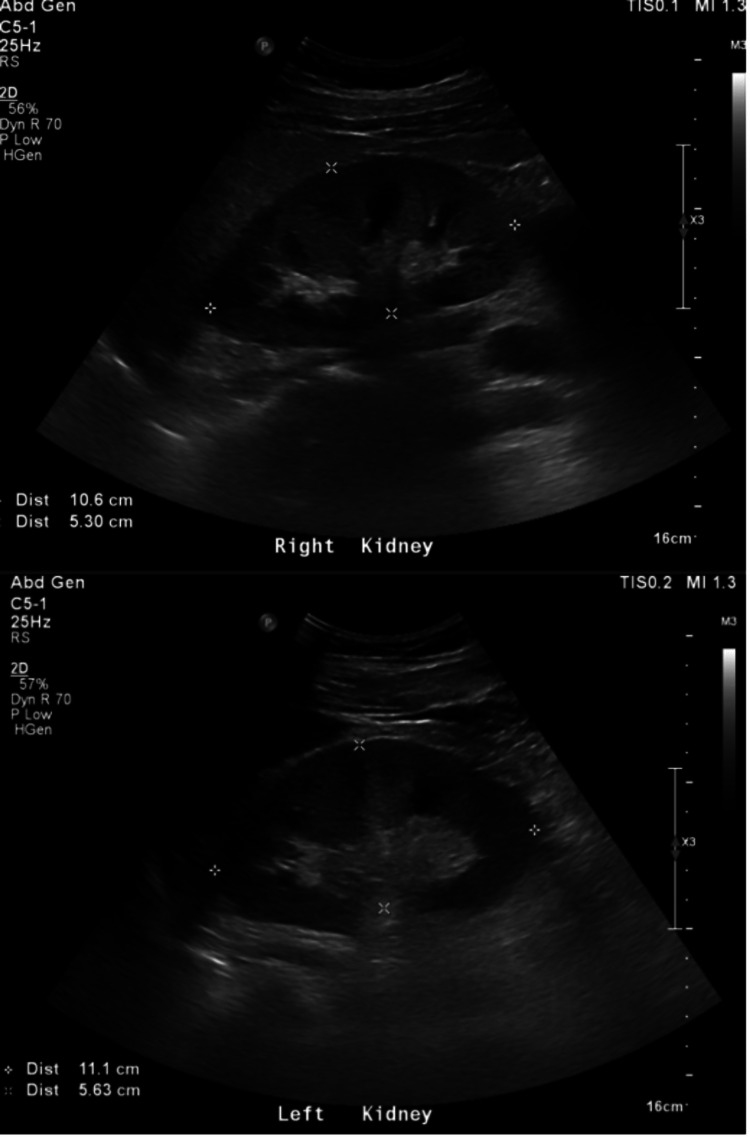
Ultrasound of the abdomen and pelvis shows both kidneys appear normal in shape, size, and echogenicity.
